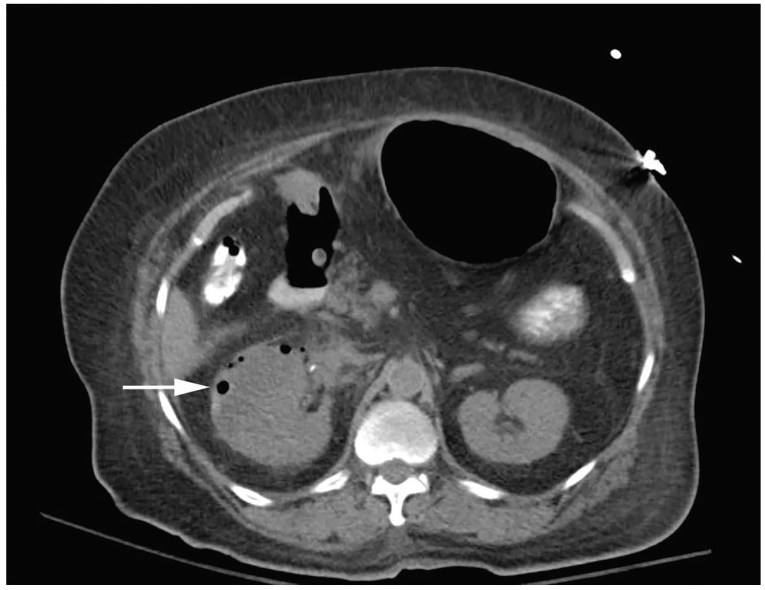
Computed tomography of the abdomen and pelvis showing an enlarged right kidney with perinephric stranding and air in the renal cortex (white arrow) consistent with emphysematous pyelonephritis.
